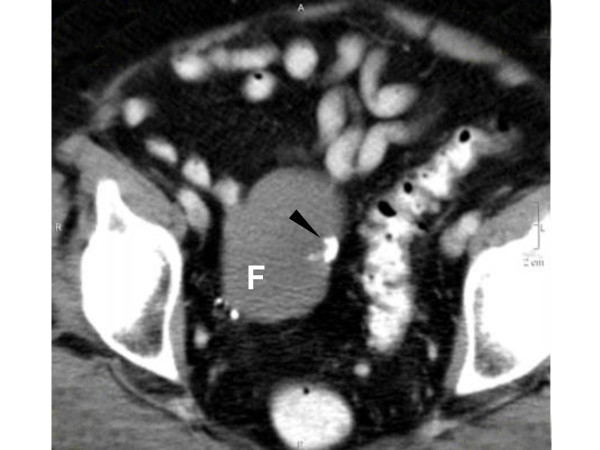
Pelvic CT demonstrating 7 cm fibrothecoma (F) in the upper right pelvis and associated calcification (arrow). No ascites or bowel dilation is present. Uterus and left ovary are surgically absent in this 75 year-old patient.
